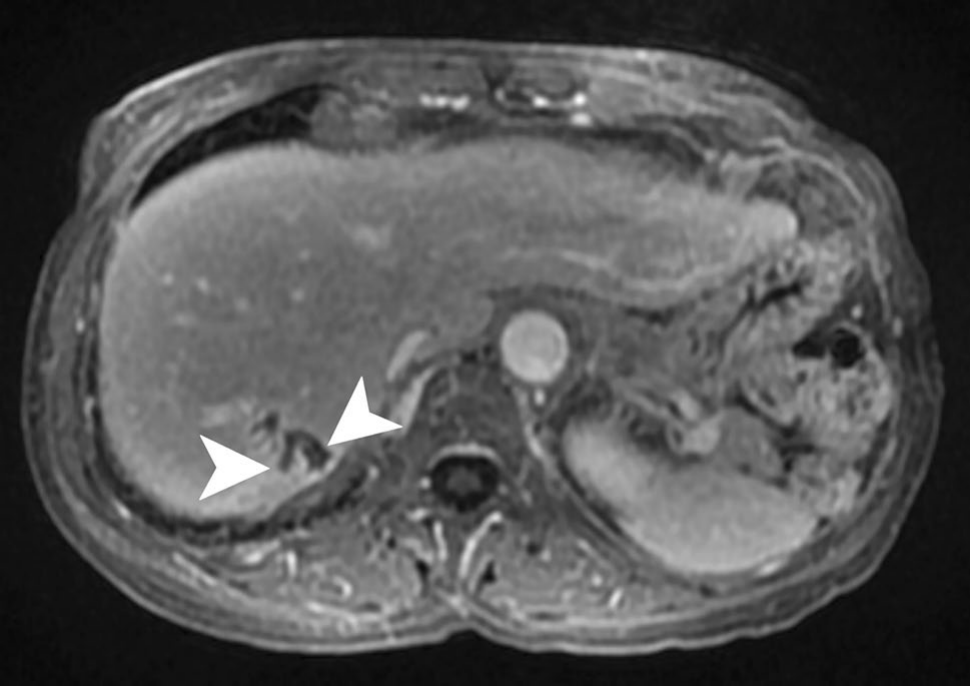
Clonorchiasis in a 74-year-old female patient with recurrent cholangitis. Axial contrast-enhanced fat-suppressed T1-weighted image shows intrahepatic bile ducts dilatation in segment VII reaching the subcapsular zone (white arrowheads)
